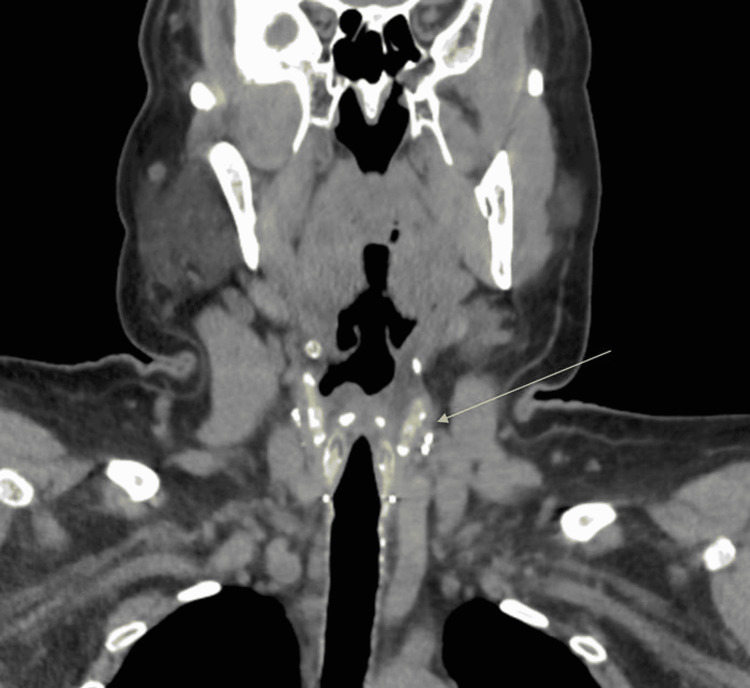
CT of the neck without contrast indicating post-treatment changes related to prior thyroidectomy, with no residual present thyroid tissue or any pathologically enlarged lymph nodes. Arrow pointing to the paratracheal surgical clips after prior thyroidectomy. CT: computerized tomography
